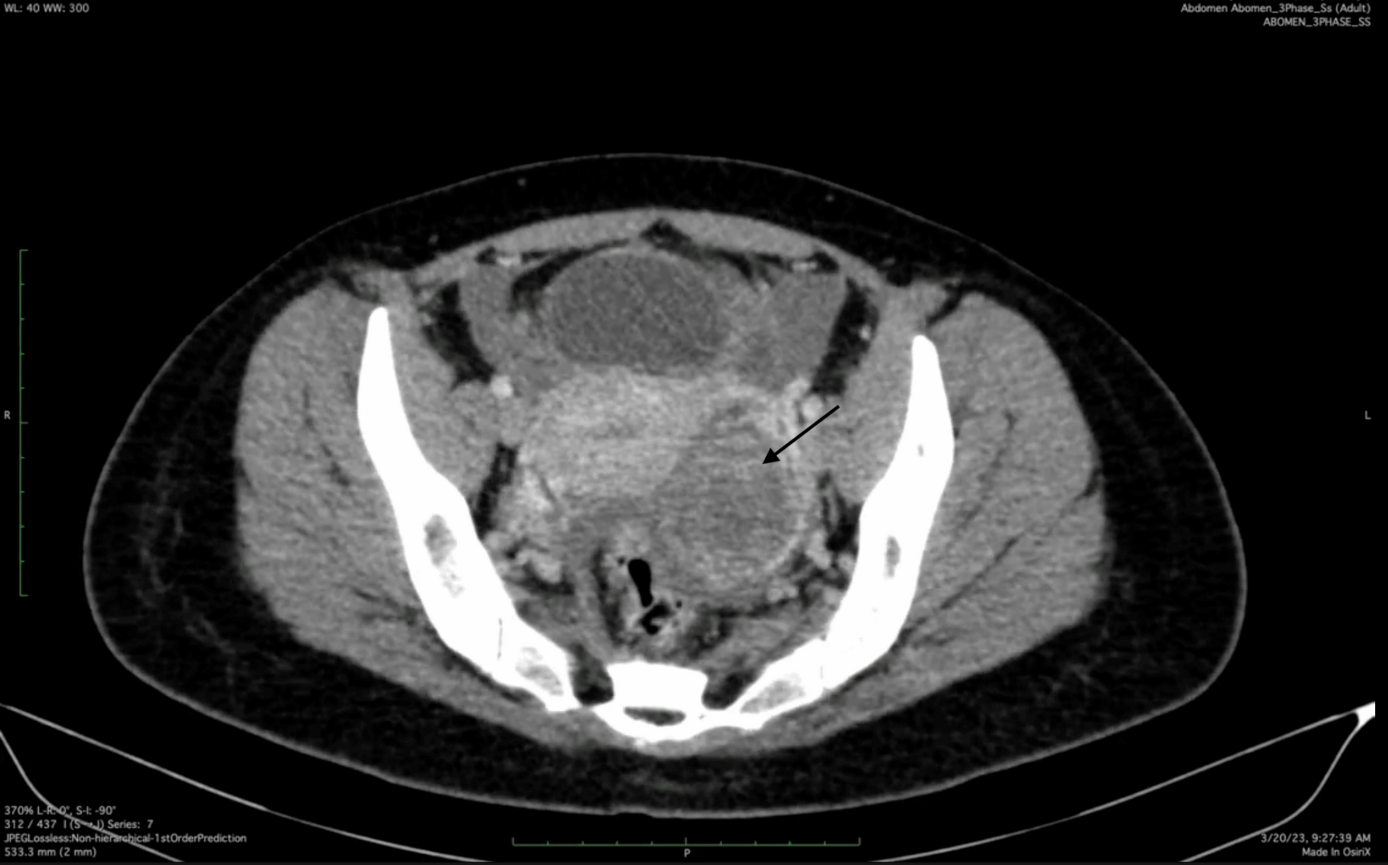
Computed tomography (CT) image taken on hospital day 3, showing an adnexal cyst with peripheral ring enhancement (arrow), suspicious for a tubo-ovarian abscess
